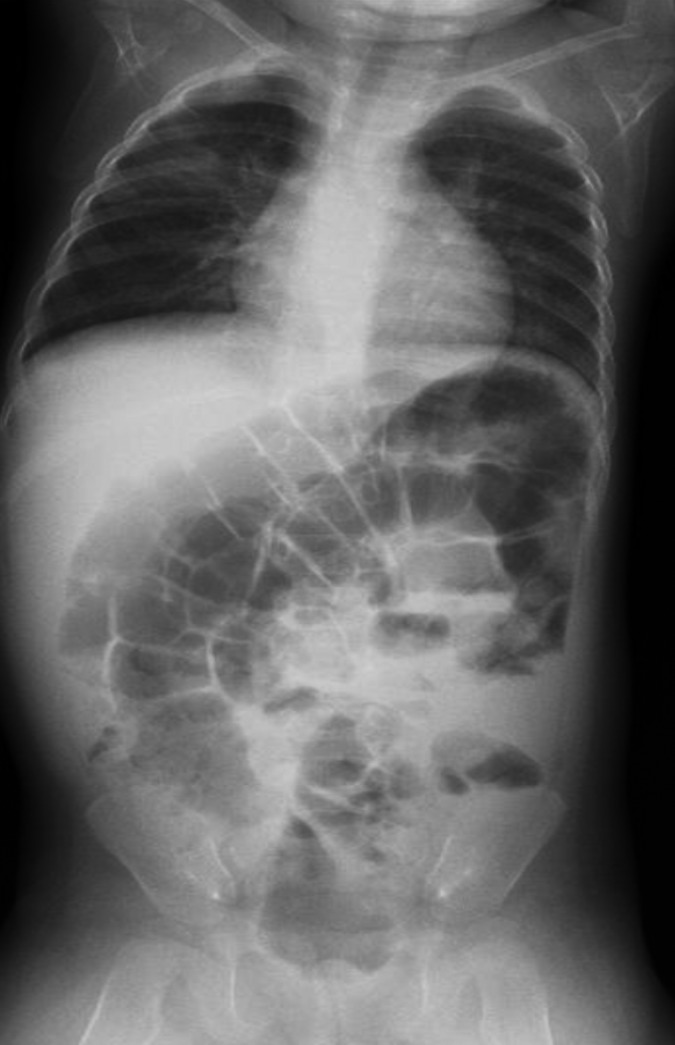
Plain abdominal radiograph at age 12 mo showing enlarged bowel similar to findings of ileus
